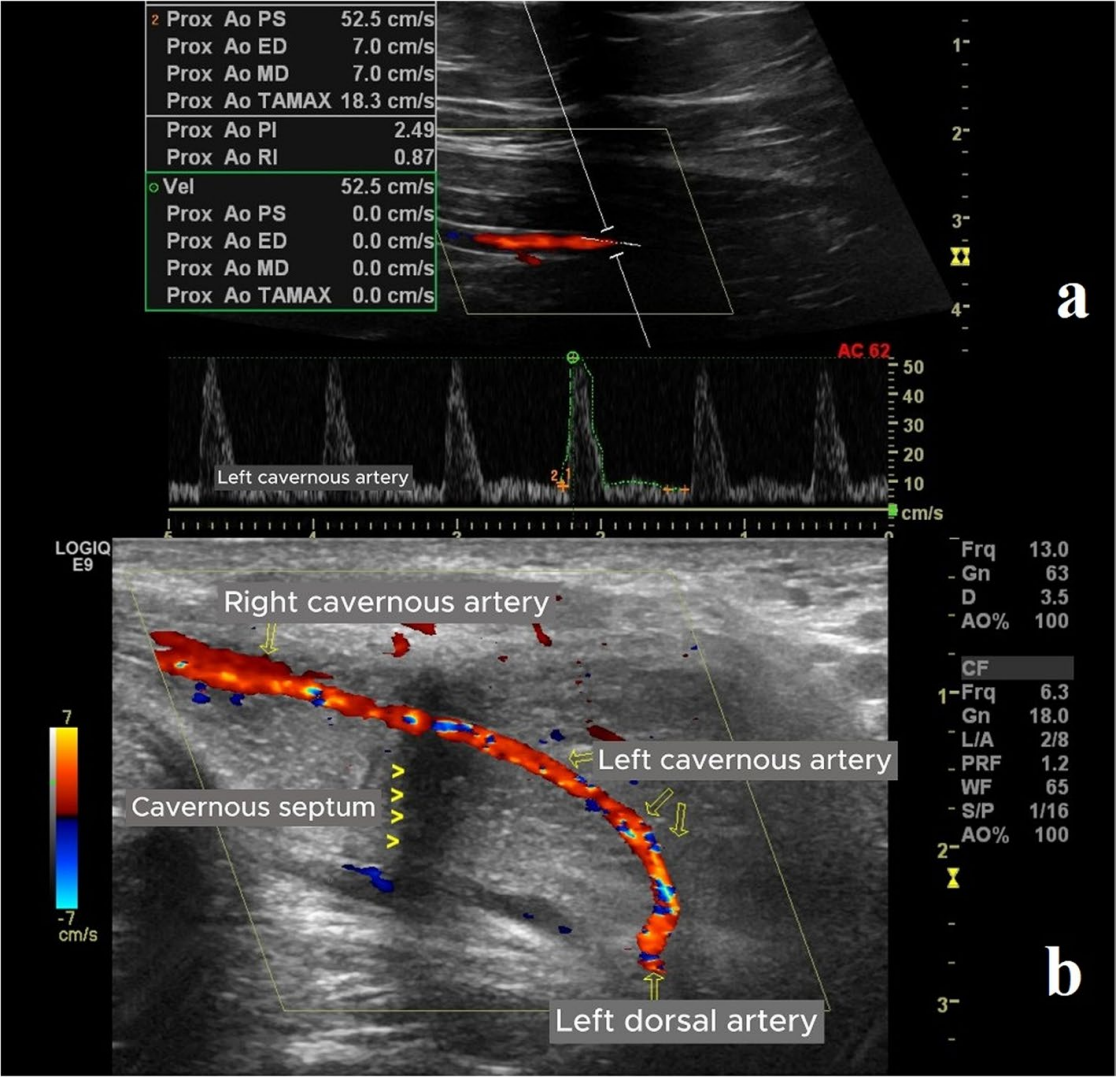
a, b) PCDU images showing the measurement of penile vascular hemodynamic parameters (PSV, EDV, and RI) after penile revascularization. In the postoperative PCDU, RI increased in correlation with IIEF scores. PS: Peak systolic velocity, ED: End-diastolic velocity, RI: Resistive index, PI: Pulsatility index, MD: Mid-diastolic velocity, PCDU: Penile color Doppler ultrasonography
